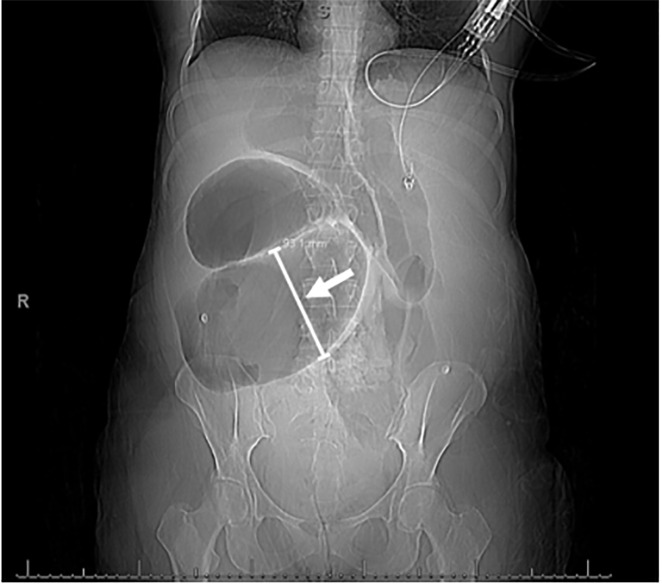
Coronal view of the patient’s scout film prior to computed tomography shows acute midgut volvulus. Loops of proximal small bowel were severely dilated (arrow), measuring 9.31 centimeters in the central anterior abdomen.
